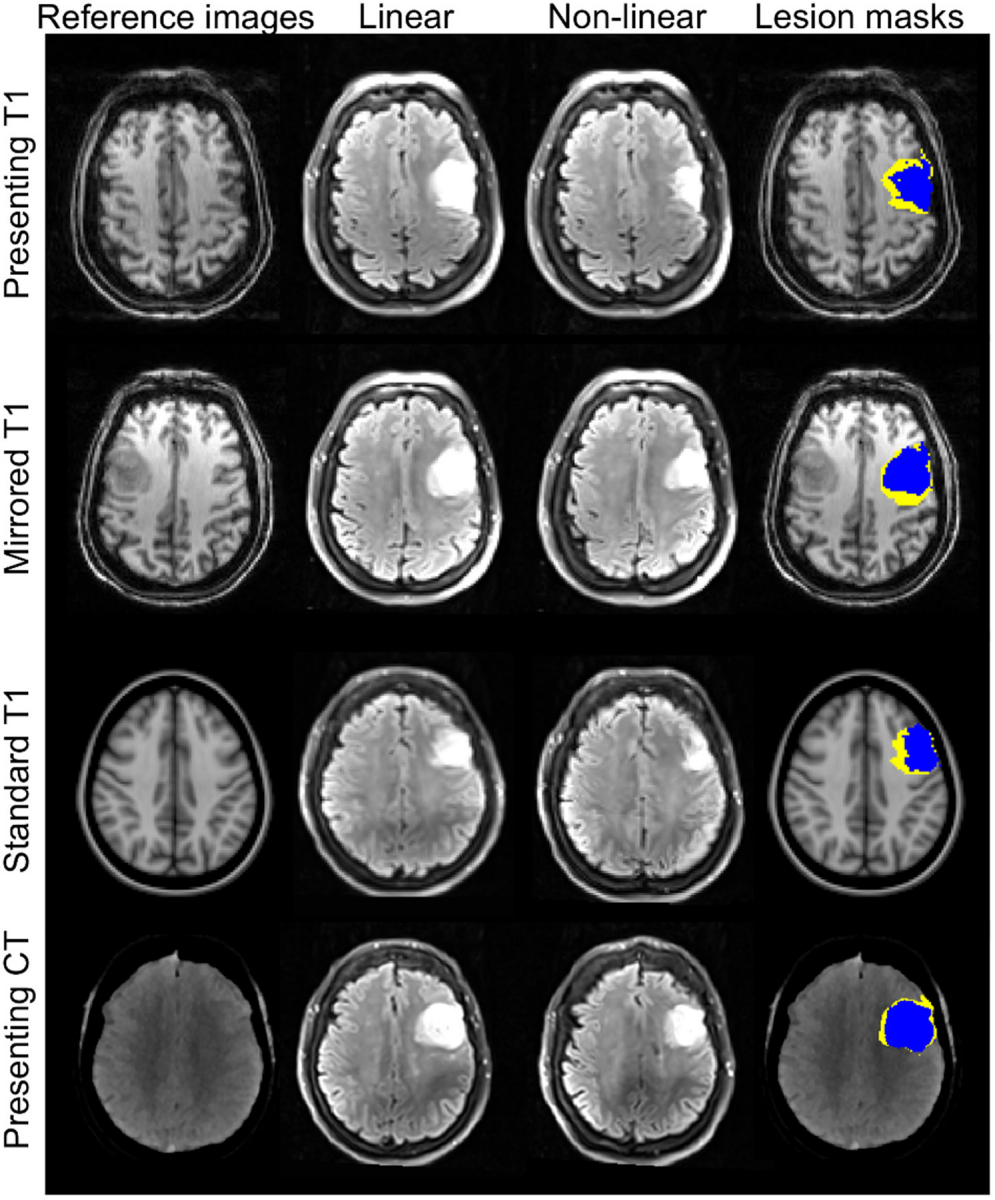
Representative imaging from a single patient. T2-weighted fluid attenuated inversion recovery imaging at 1 week and the associated infarct masks were registered to the different reference images. Imaging is displayed in the reference image space, accounting for the difference in image orientations. The anatomic distortion can be quantified as the difference between the linearly (yellow) and nonlinearly (blue) registered masks in the reference image space. CT indicates computed tomography.
